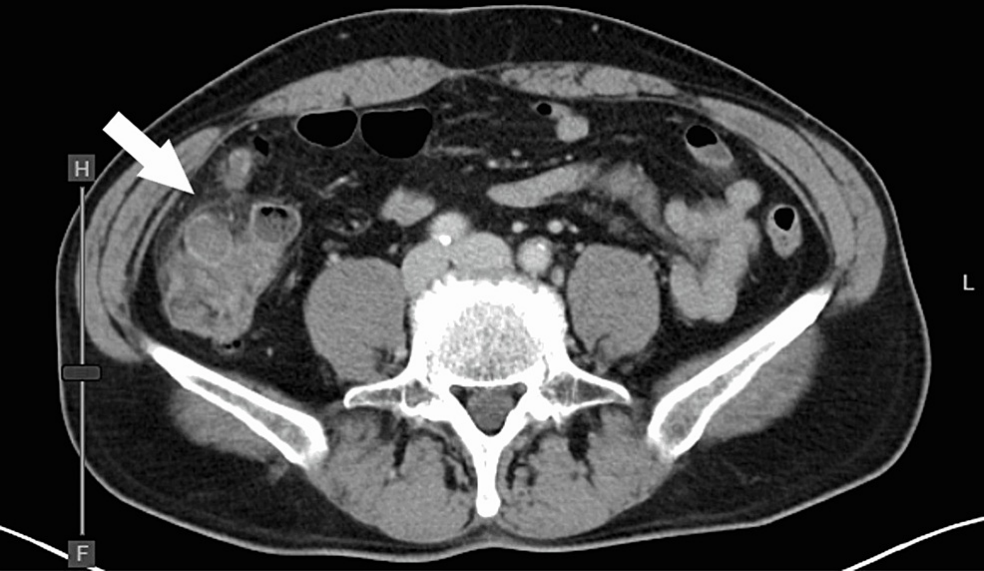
Abdominal contrast-enhanced CT scans, axial view Diverticula and pericolic fat stranding were confirmed in the cecum (arrow).
